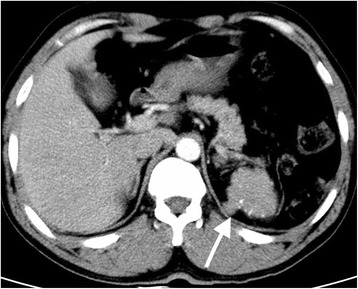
CT showing a huge tumour located in the splenic fossa and the tumour invading into the splenic flexure of the colon
